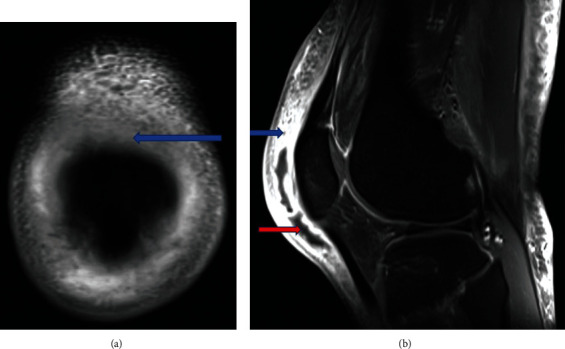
(a) MRI coronal view reveals an area of hypodensity in the prepatellar bursa indicating bursitis (blue arrow). (b) Sagittal T1 view reveals prepatellar bursitis (blue arrow) and abscess (red arrow).
